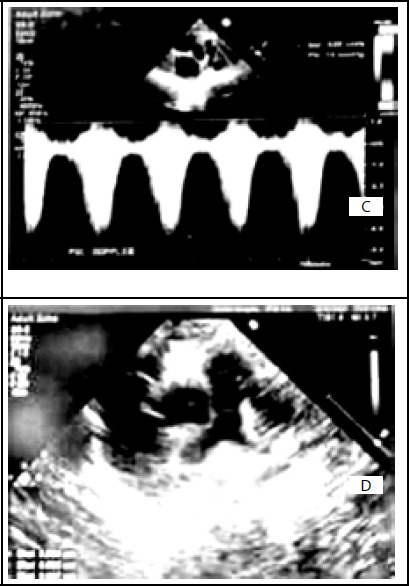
Echocardiography of congenital tricuspid atresia type IB with ASD and VSD. (C); Doppler study of pulmonary valve. Increased gradient across the stenotic pulmonary valve. (D); Parasternal short axis view. The pulmonary artery segment from the valve till the bifurcation.
